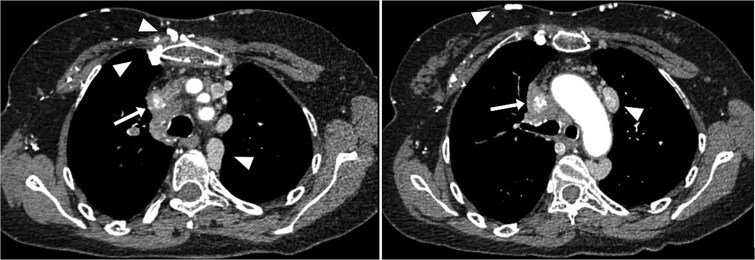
Axial CT scan with contrast injection showing parietal thickening of the SVC leading to luminal narrowing (arrow). Collateral venous circulation is also noted, indicating obstructed venous drainage and thus superior vena cava syndrome (arrowheads).
